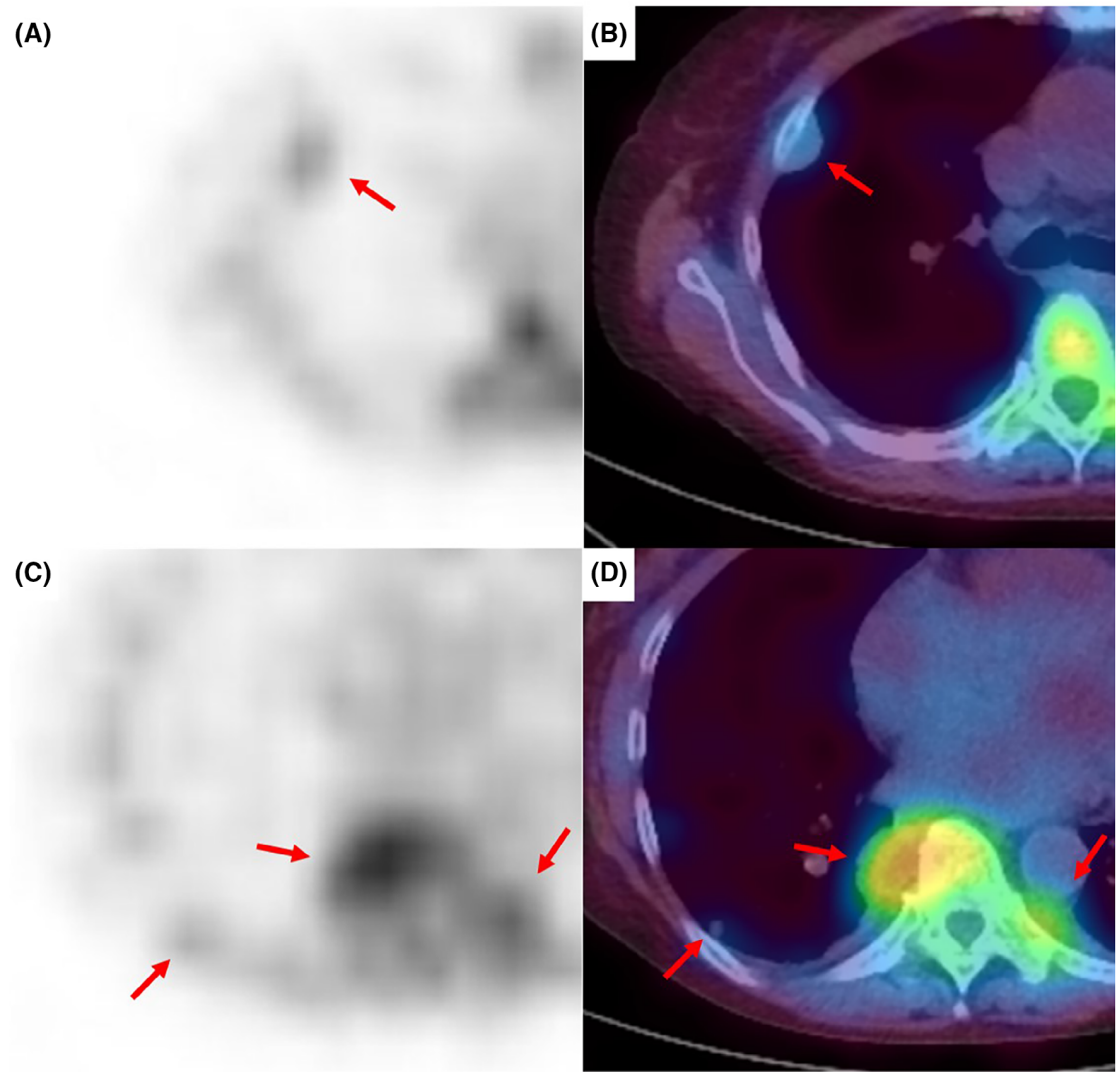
Images of ^111^In‐bone marrow scintigraphy show accumulation of ^111^In in the intrathoracic nodular lesions which locate in the right pleura (A, B), the bilateral paraspinal (C, D), and the right lower lobe (C, D).
